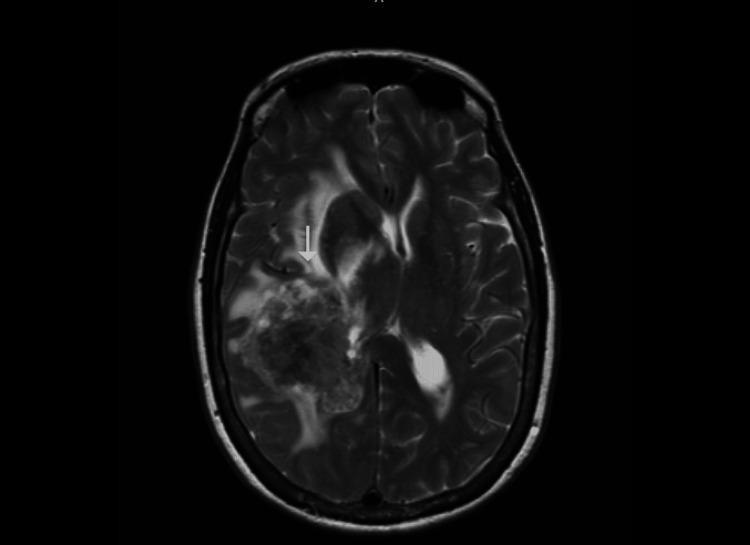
MRI of the brain with and without contrast (T2 sequence) showing an approximately 5.8 cm intra-axial heterogeneous mass (white arrow) in the right frontotemporal lobe, with extensive surrounding edema and local mass effect on the ventricles and midline shift.
